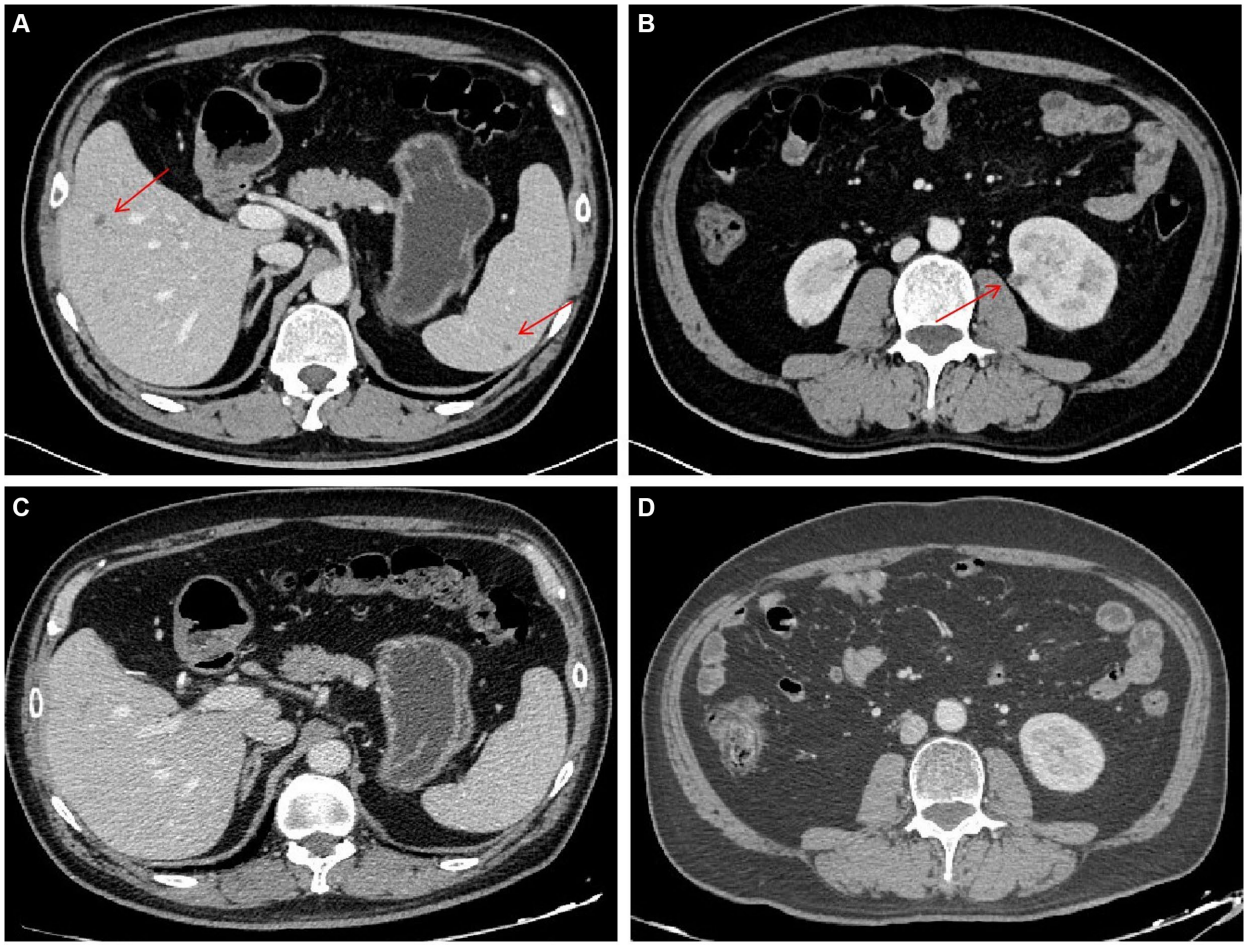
Contrast-enhanced CT images of chronic disseminated candidiasis. (A,B) Day 39, multiple small abscesses in the liver, spleen and both kidneys (the red arrows). (C,D) Day 171, abscesses almost disappeared.
